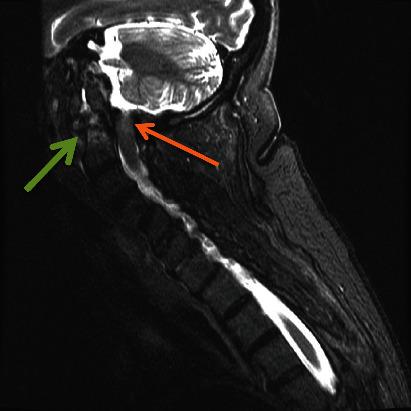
Cervical MRI (STIR) showing increased STIR signal in the spinal cord at the C1 level (orange arrow) and odontoid fracture (green arrow).
